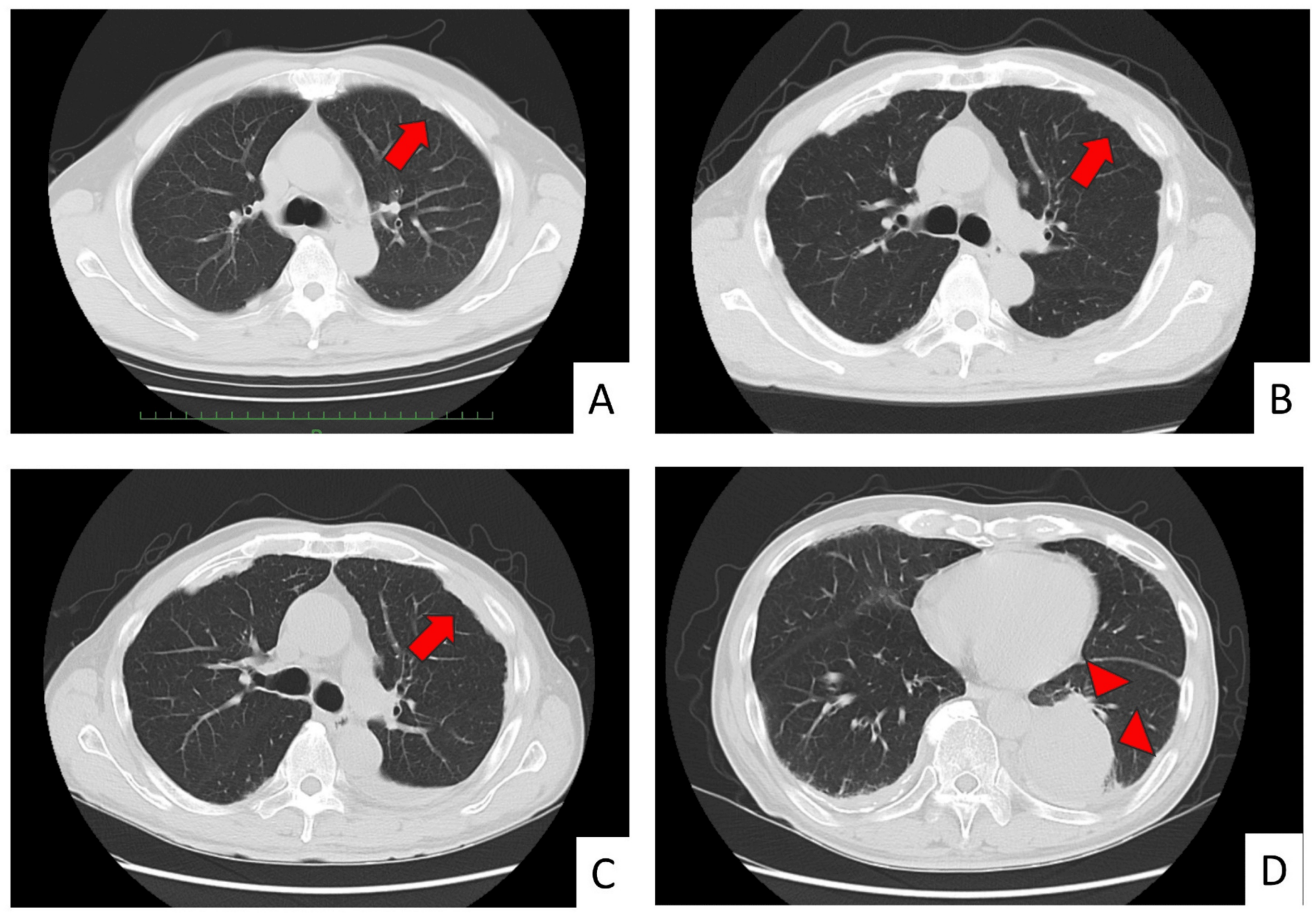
Chest computed tomography. (A) Twelve years prior (year X-12): a small nodule around the left third intercostal space (arrow). (B) Three years prior (year X-3): a pleural plaque between the left third and fourth intercostal spaces (arrow). (C) Year X: pleural thickening can be seen centered at the left third/fourth intercostal space (arrow). Compared to the lesions in the image from 12 years earlier, the lesion in the left third/fourth intercostal space shows a tendency to increase in size over time. (D) Year X (same as C): a mass measuring approximately 68 mm in the left lower lobe (arrowheads).
